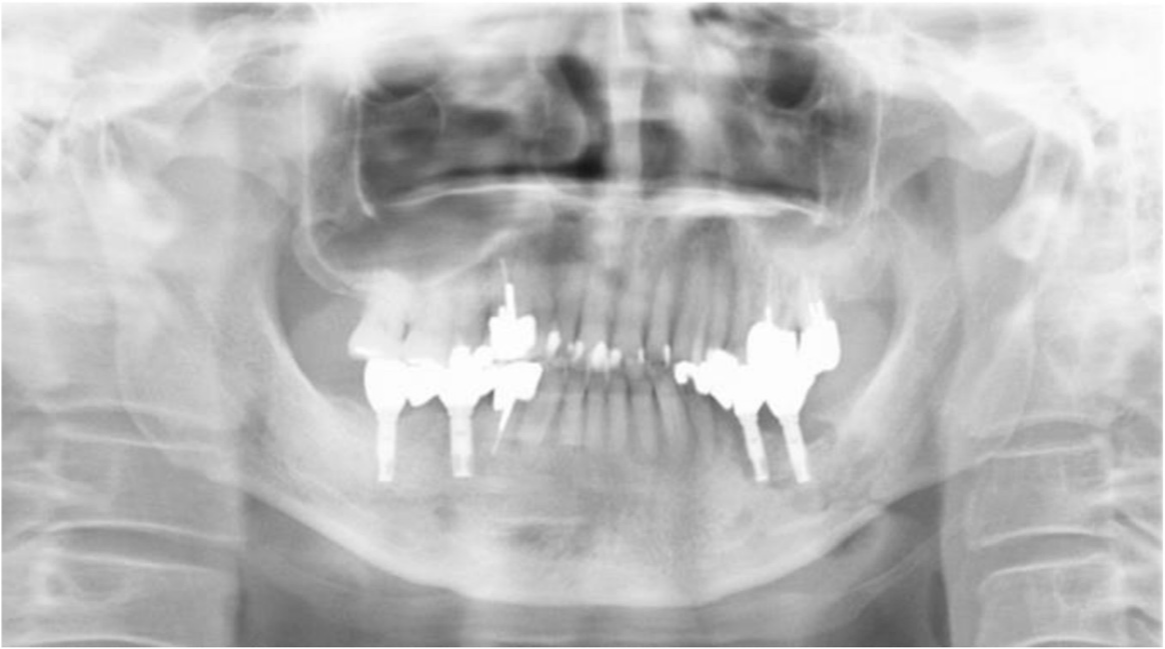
Panoramic X-ray image at the first visit
